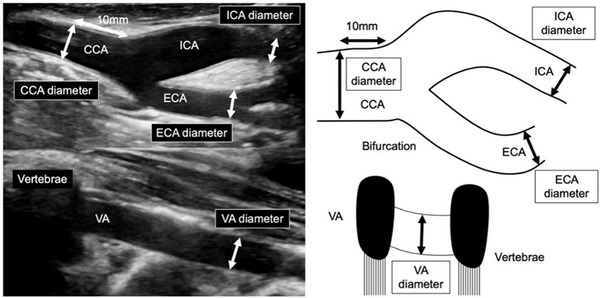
Carotid ultrasonography measurement of cervical artery diameters. CCA indicates common carotid artery; ECA, external carotid artery; ICA, internal carotid artery; and VA, vertebral artery.
